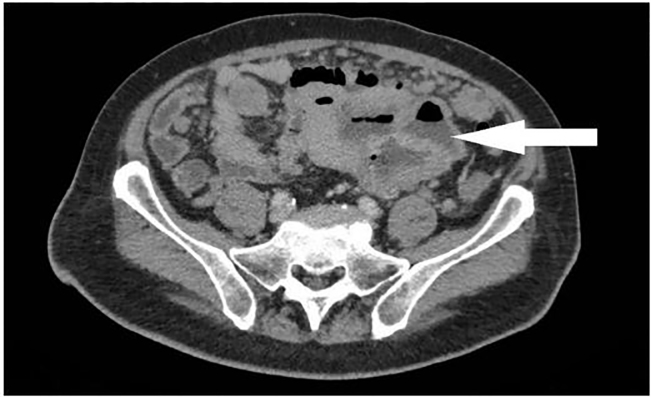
Computed tomography of the abdomen and pelvis showing a centrally necrotic mass of 9.3.x 11.6 × 9.3 cm that was closely related to the small bowel loops as indicated by the white arrow, later determined to be a gastrointestinal stromal tumor.
